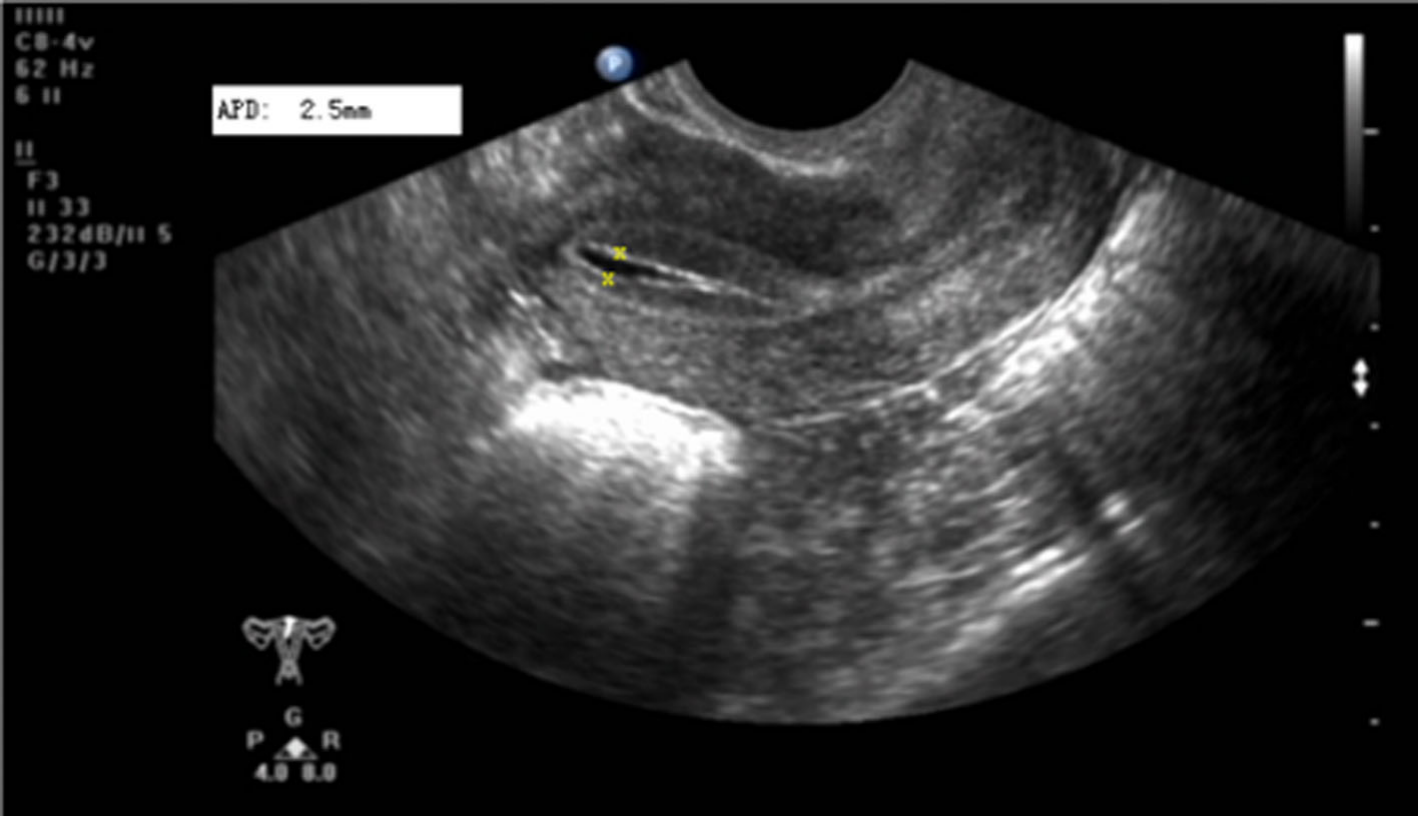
Endometrial cavity fluid (ECF). Fluid accumulation in the uterine cavity detected by transvaginal ultrasound in a sagittal view (A--P diameter 2.5 mm) in a patient on the day of oocyte retrieval.
